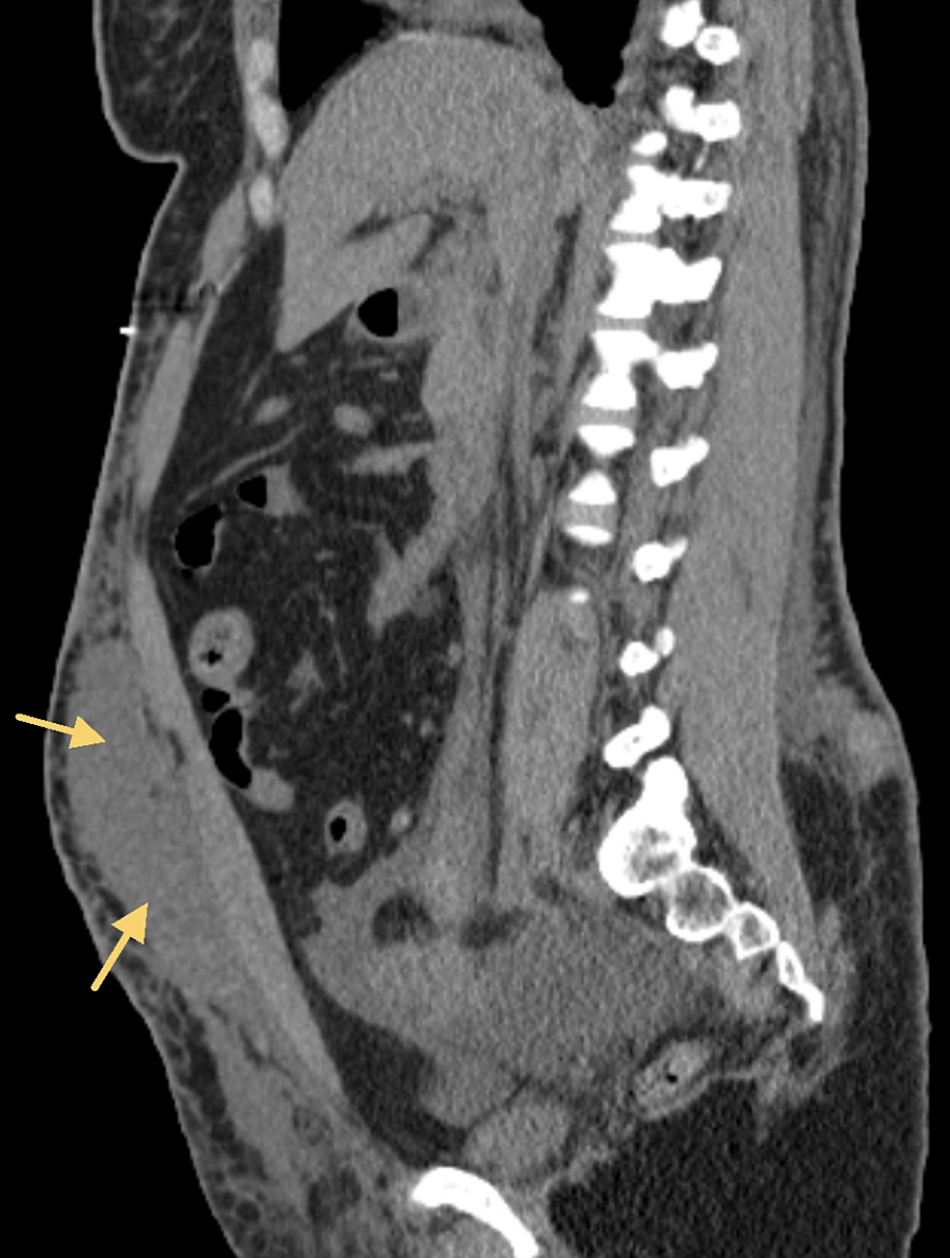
Computed tomography sagittal view of hematoma in abdominal and pelvic cavities bilaterally (arrows).
